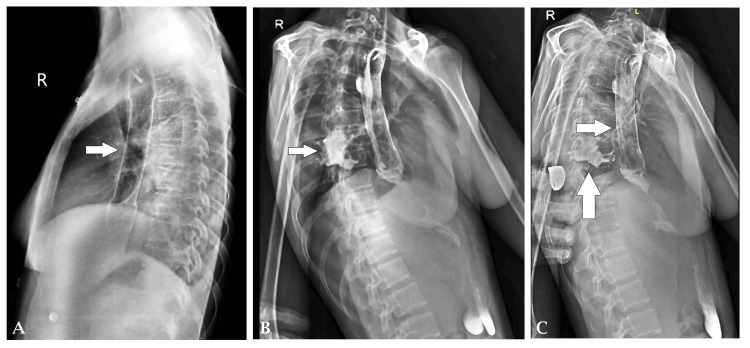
(A) A lateral chest X-ray showing the placement of the esophageal stent. (B) Contrast study showing leak of contrast from the esophagus to the right bronchus with a tract filled with contrast. (C) Contrast study showing leak of contrast in the cavity in the right lower lobe
